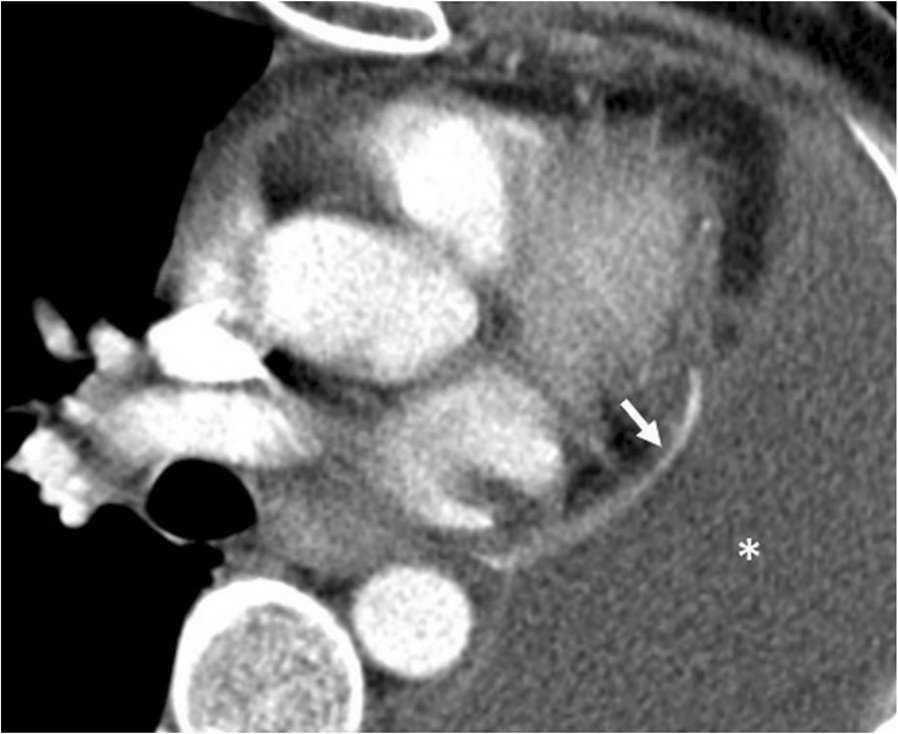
A 50-year-old man with lung cancer. Axial contrast-enhanced CT image demonstrates a linear hyperdense pericardial patch (arrow). Large amount of fluid (asterisk) in left pneumonectomy cavity is also noted
